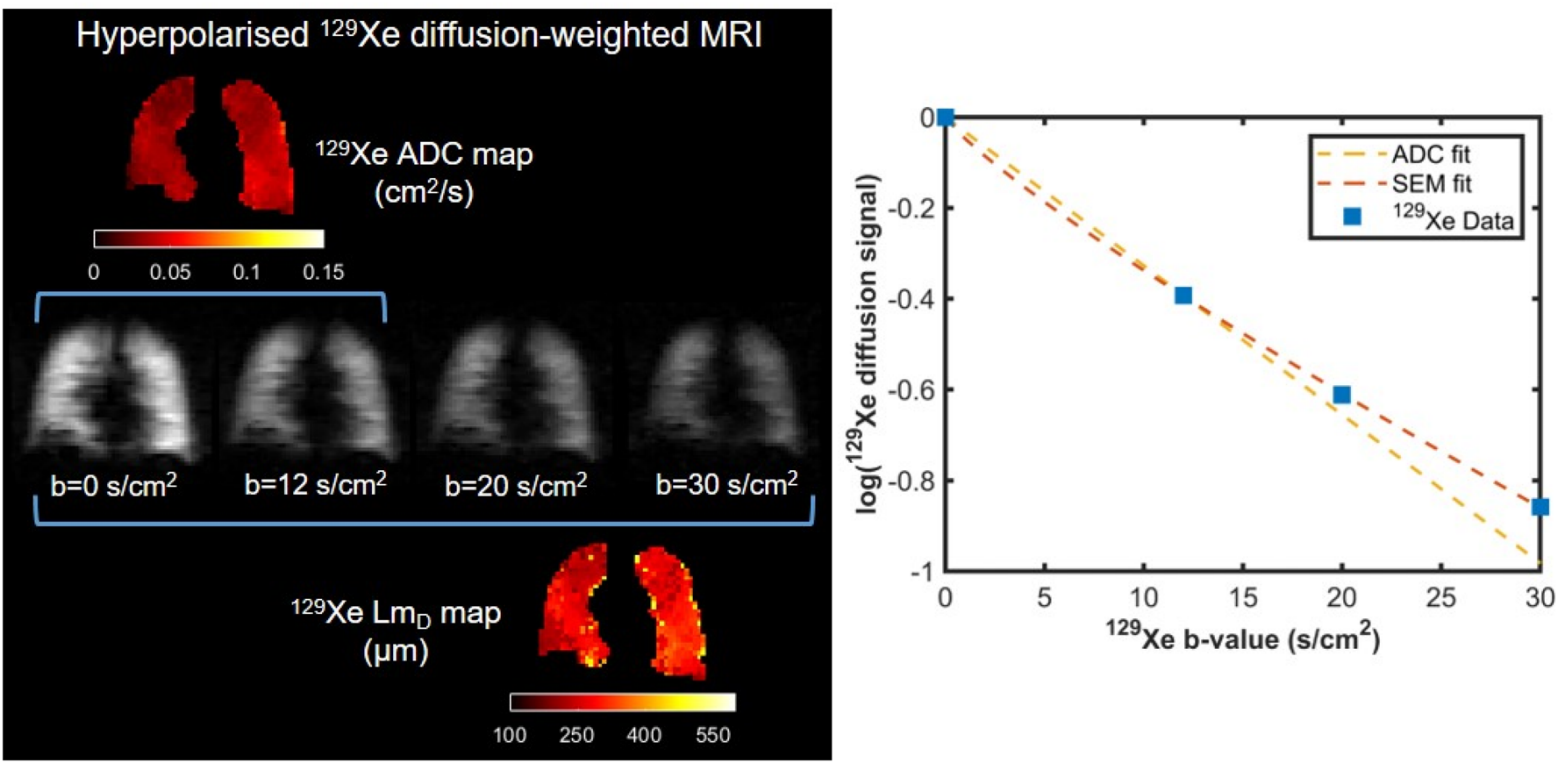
Example hyperpolarised ^129^Xe diffusion-weighted (DW) MRI data from one volunteer. (Left) Maps of ADC and *Lm*_*D*_ values calculated from ^129^Xe DW-MRI. (Right) Representative global ^129^Xe signal as a function of b-value, ADC- and SEM-fits obtained for the same volunteer.
